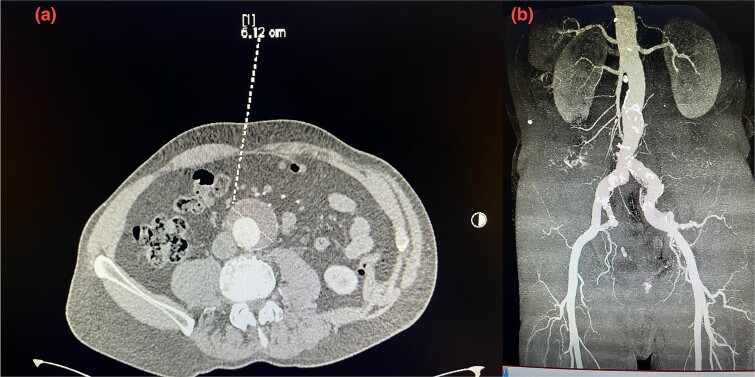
(a) Pre-operative axial CT scan of the infrarenal aorta showing an aneurysm (arrow) measuring 6.10 cm. (b) Initial CTA scan. A detailed anatomy of the bilateral iliac arteries is presented, and the axial image of the CTA demonstrated bilateral common iliac artery ectasia, measuring 2.9 cm on the right side and 2.4 cm on the left side.
